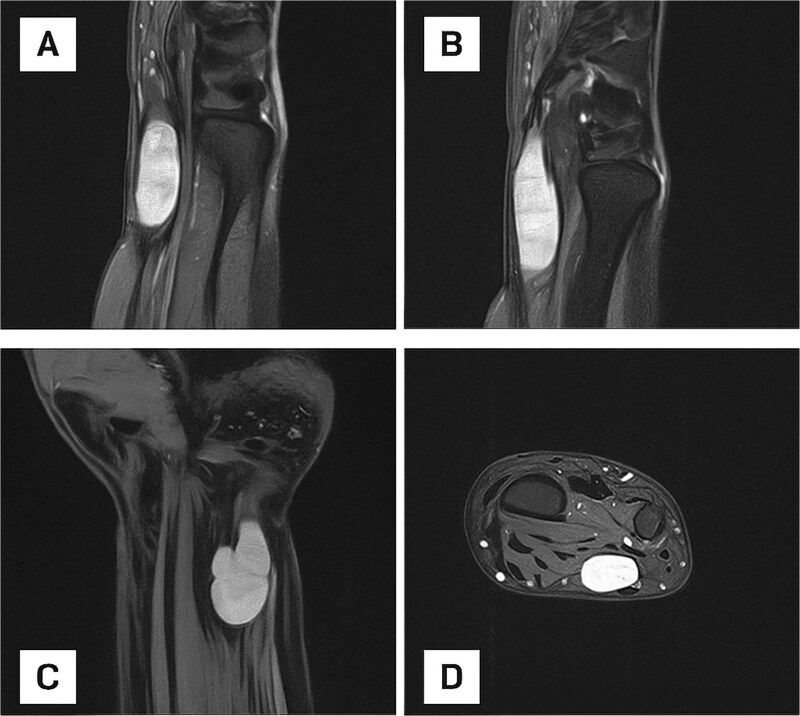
(A) Sagittal MRI scan of the distal forearm demonstrating a well-defined, hyperintense lesion on the ulnar aspect, suggestive of a fluid-rich or soft tissue mass. (B) Sagittal MRI scan depicting an elongated, well-circumscribed hyperintense lesion with no evidence of bone involvement or perilesional edema. (C) Coronal MRI scan showing a lobulated mass, raising suspicion for a nerve sheath tumour or multilobulated ganglion cyst. (D) Axial MRI view confirming an encapsulated lesion within the deep soft tissue of the distal forearm.
